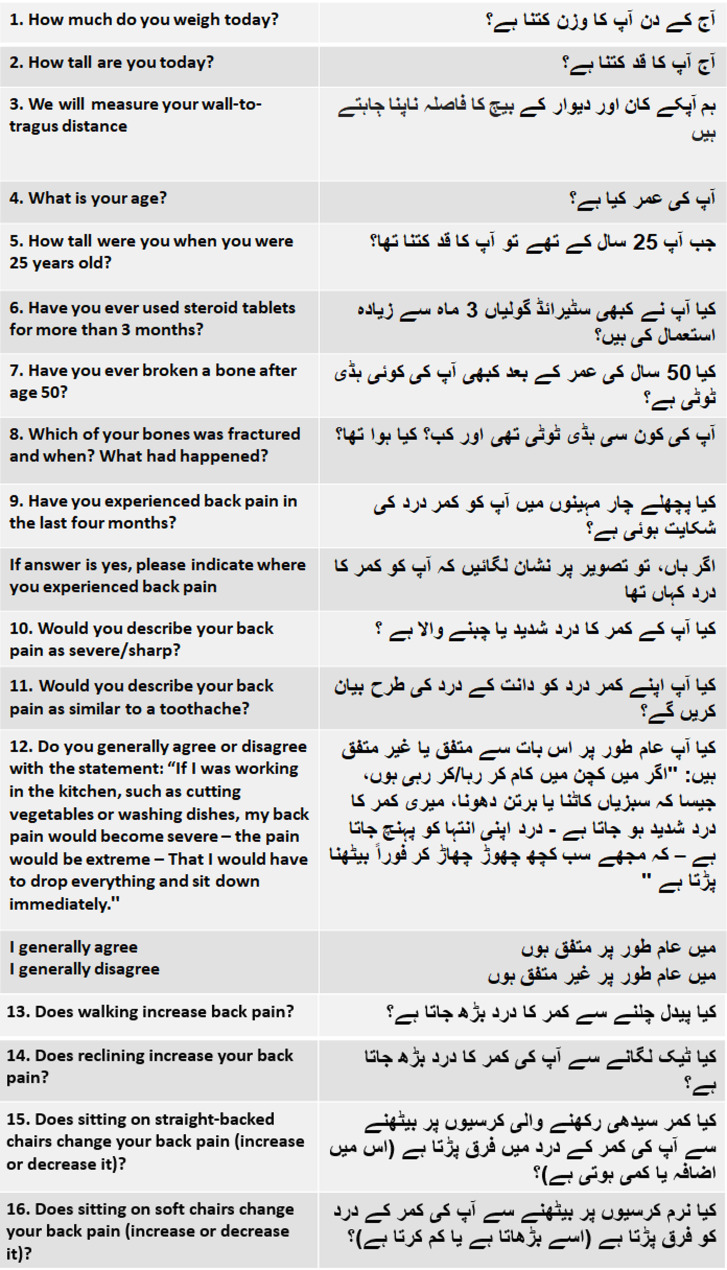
Urdu-Vfrac questions to help identify whether a spinal X-ray should be recommended for possible vertebral fragility fracture
